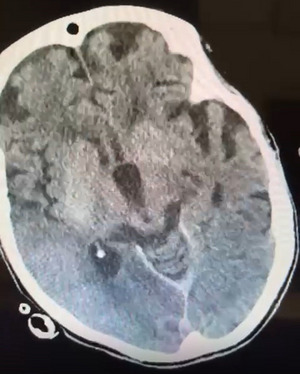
Type 2 hemorrhagic infarction in the brain, computed tomography scan taken after mechanical thrombectomy in a patient with distal occlusion of MCA M1.
